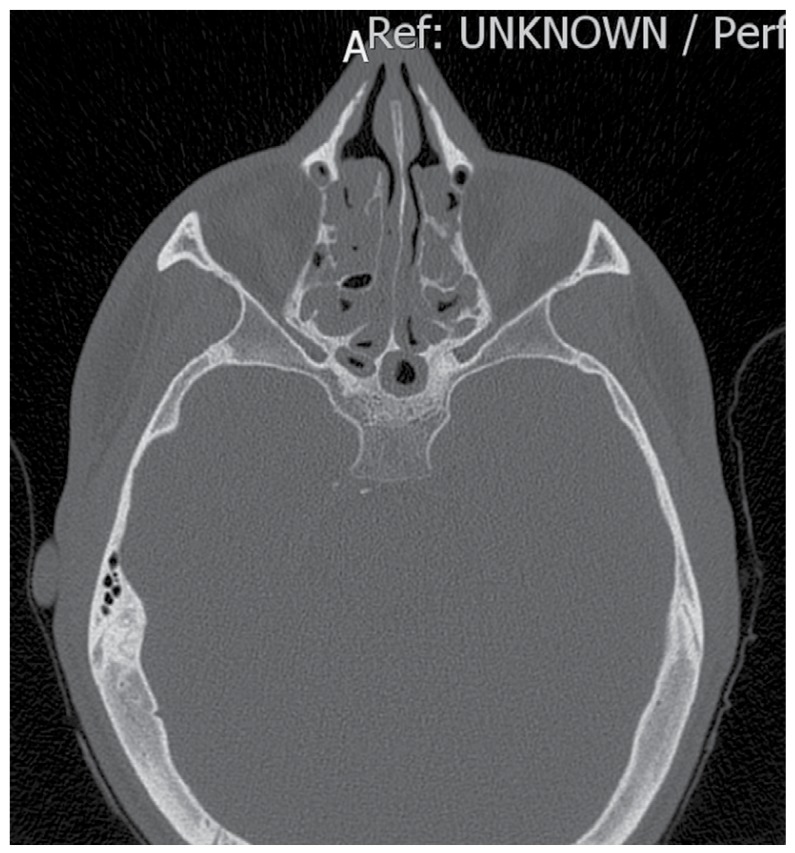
Axial CT scan of the sinuses showing sphenoid sinus hypoplasia in a 25-year-old patient with cystic fibrosis.
